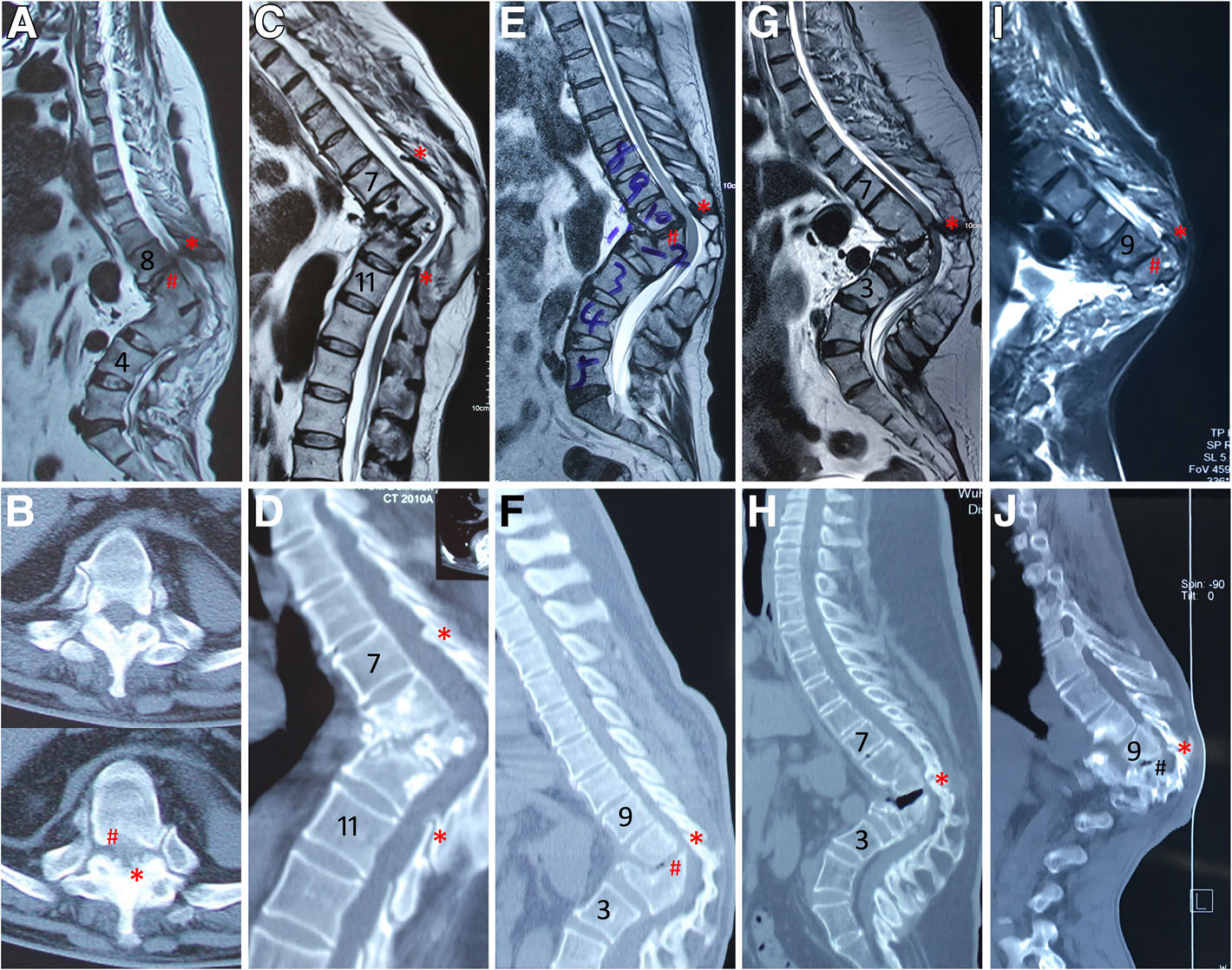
Causes of late-onset neurological deficits according to the lesions. a, b Preoperative magnetic resonance image (MRI) and computed tomography (CT) scanning demonstrate severe angular kyphosis, “buckling collapse” from T9 to L3, intervertebral disc herniation and calcified ligamentum flavum at T8/9, and severe spinal cord compression. c, d Preoperative MRI and CT scanning demonstrate severe angular kyphosis from T8 to T10, calcified ligamentum flavum at T7/8 and T10/11, and severe spinal cord compression. e, f Preoperative MRI and CT scanning demonstrate severe angular kyphosis, “buckling collapse” from T10 to L2, intervertebral disc herniation and calcified ligamentum flavum at T10/11, and severe spinal cord compression. g, h Preoperative MRI and CT scanning demonstrate severe angular kyphosis, “buckling collapse” from T8 to L2, calcified ligamentum flavum at T10/11, and severe spinal cord compression. i, j Preoperative MRI and CT scanning demonstrate severe angular kyphosis, intervertebral disc herniation and calcified ligamentum flavum at T9/10, and severe spinal cord compression. *Calcified ligamentum flavum at the segments adjacent to the angular kyphosis; ^#^disc herniation at the segments adjacent to the angular kyphosis
